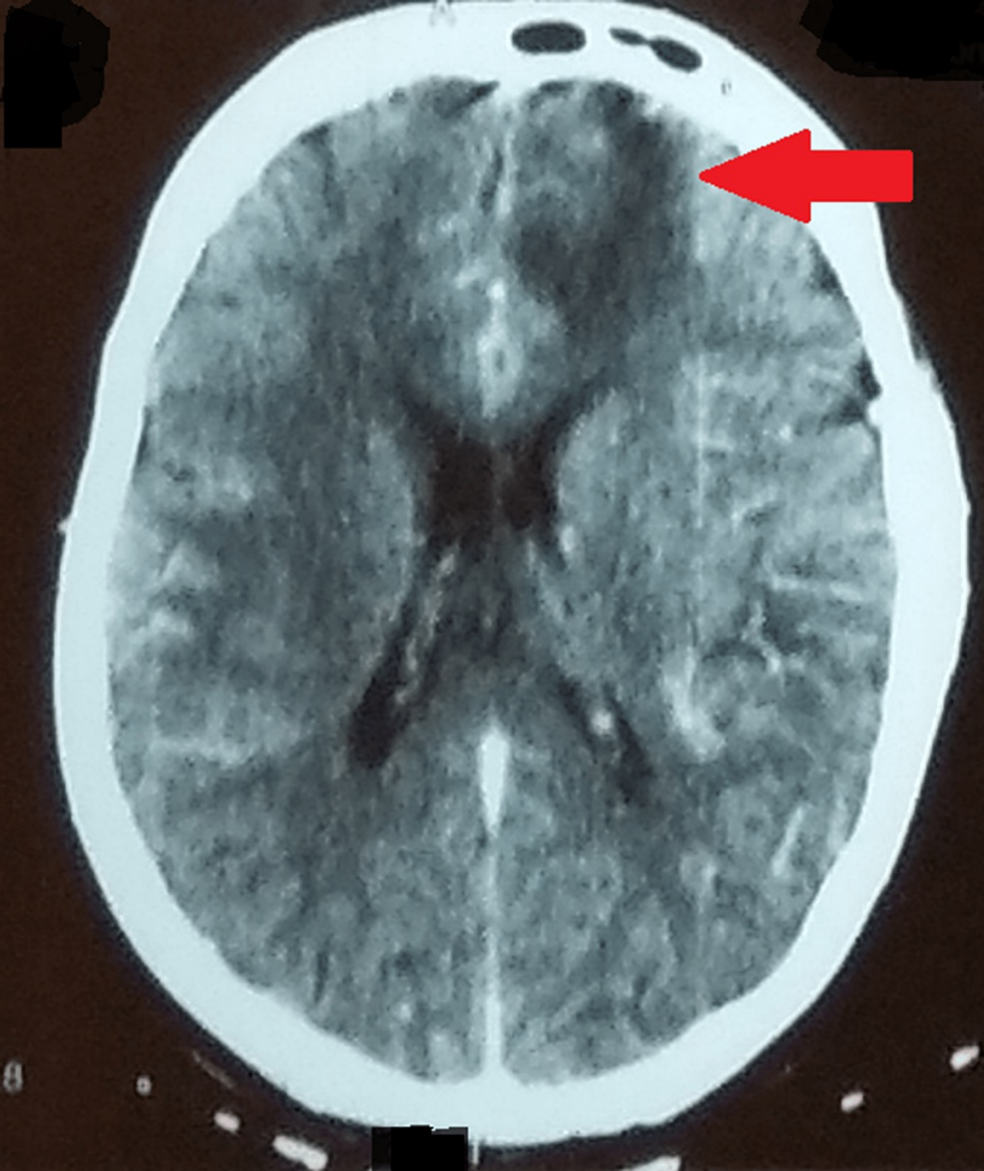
Plain CT brain showing an ill-defined isodense lesion in the left basifrontal region CT: computed tomography
